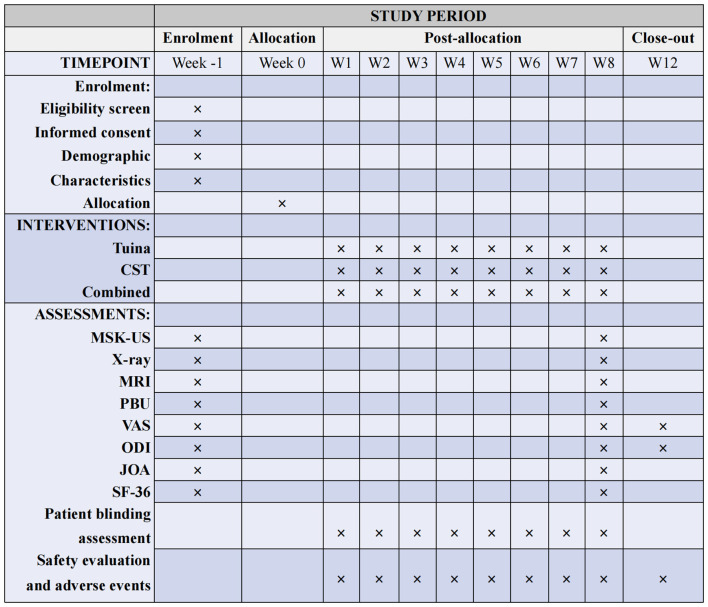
SPIRIT schedule. Enrollment, Intervention, and Assessment Schedule. CST, Core stability training group; MSK-US, Musculoskeletal ultrasound; MRI, Magnetic Resonance Imaging; VAS, Visual Analog Scale; ODI, Oswestry Disability Index; JOA, Japanese Orthopedic Association; SF-36, Short Form-36 Health Survey; PBU, Pressure Biofeedback Unit.
